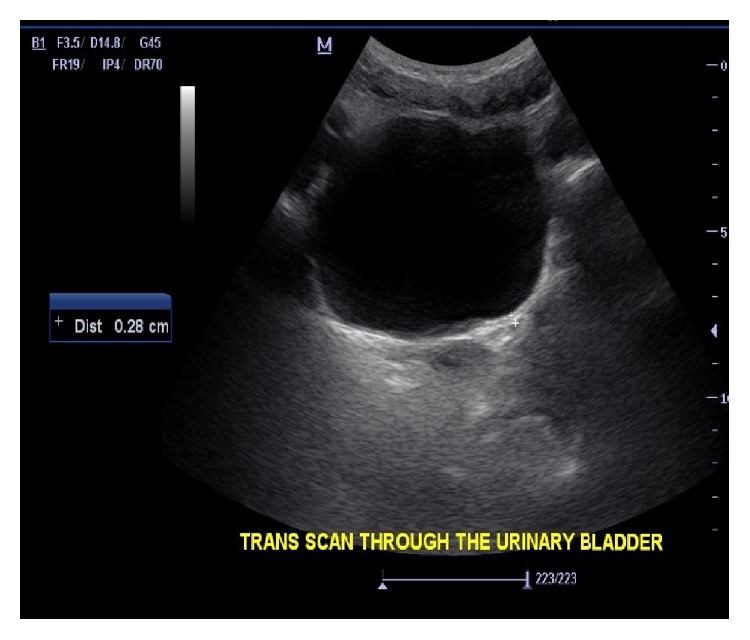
B mode ultrasound of the bladder showing a fully extended bladder with no pathology.
